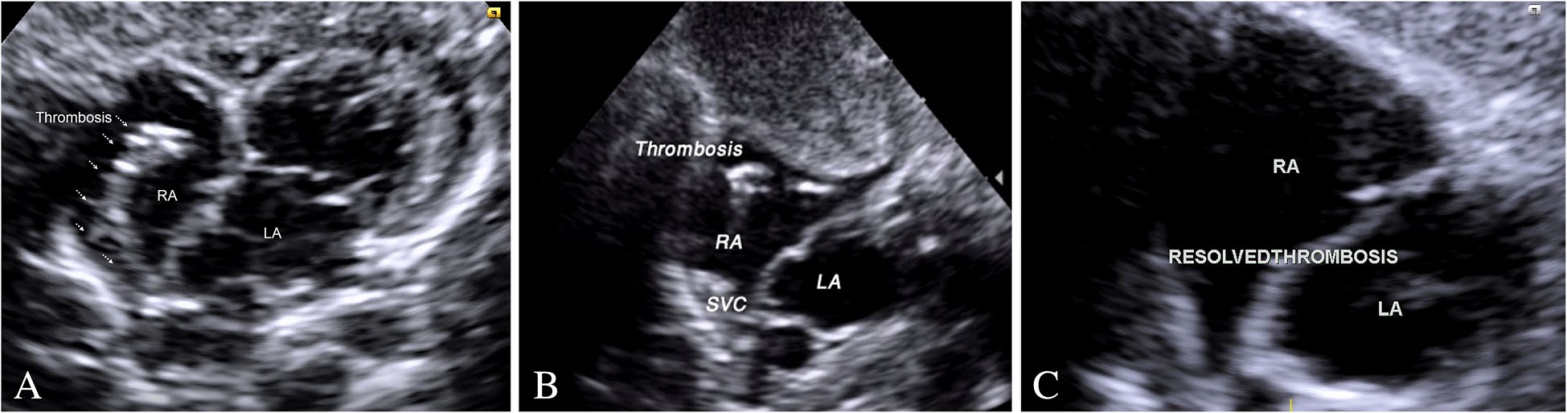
Serial echocardiography a First echocardiography, crescentic thrombotic mass in the right atrium, b After 2 months of enoxaparin treatment, c Four-month follow-up. IVC: inferior vena cava, SVS: superior vena cava, RA: right atrium, LA: left atrium, LV: left ventricle, RV: right ventricle
